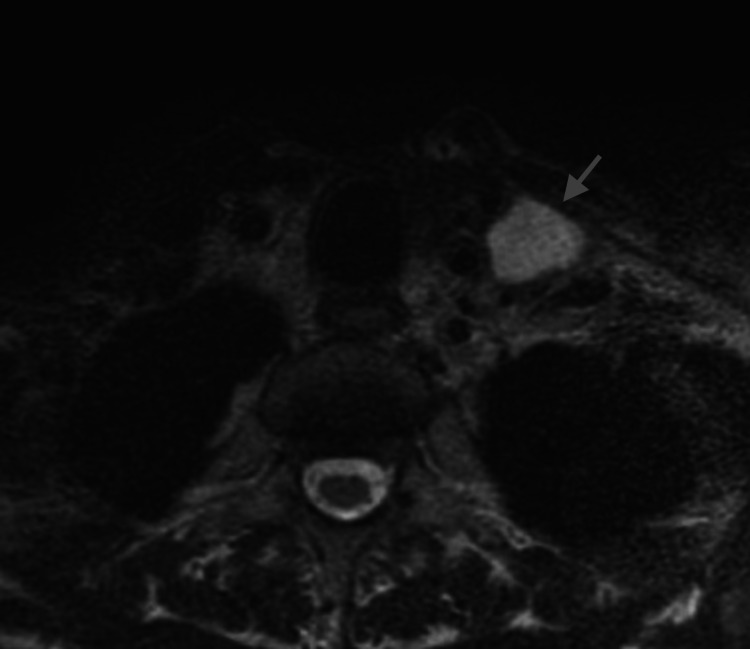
Arrow points to the chyloma on axial T2-weighted, non-enhanced MRI of the neck. The chyloma was hyperintense on T2.
